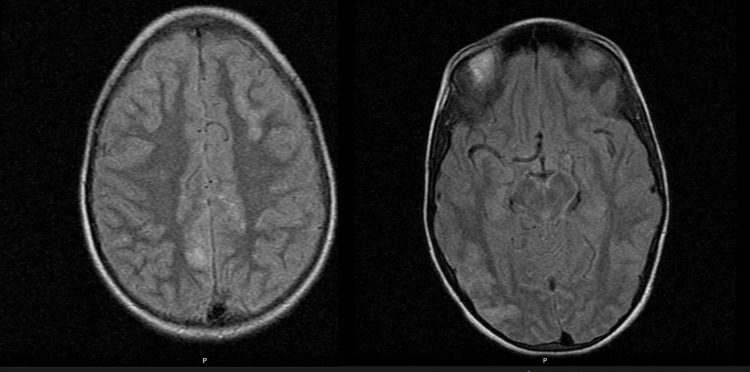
MRI findings consistent with PRES (patient 3) High-intensity cortical lesions located bilaterally on the posterior frontal regions and right-sided parieto-temporo-occipital regions.
